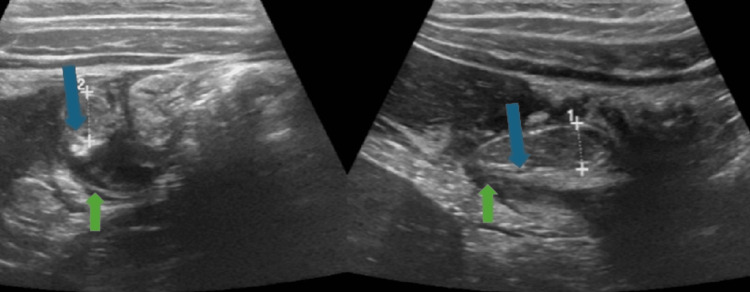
Ultrasound showing subhepatic appendix measuring 8.4 mm in diameter and minimal free fluid in the subhepatic region Blue arrow: Subhepatic appendix; Green arrow: free minimal fluid in the subhepatic region.
